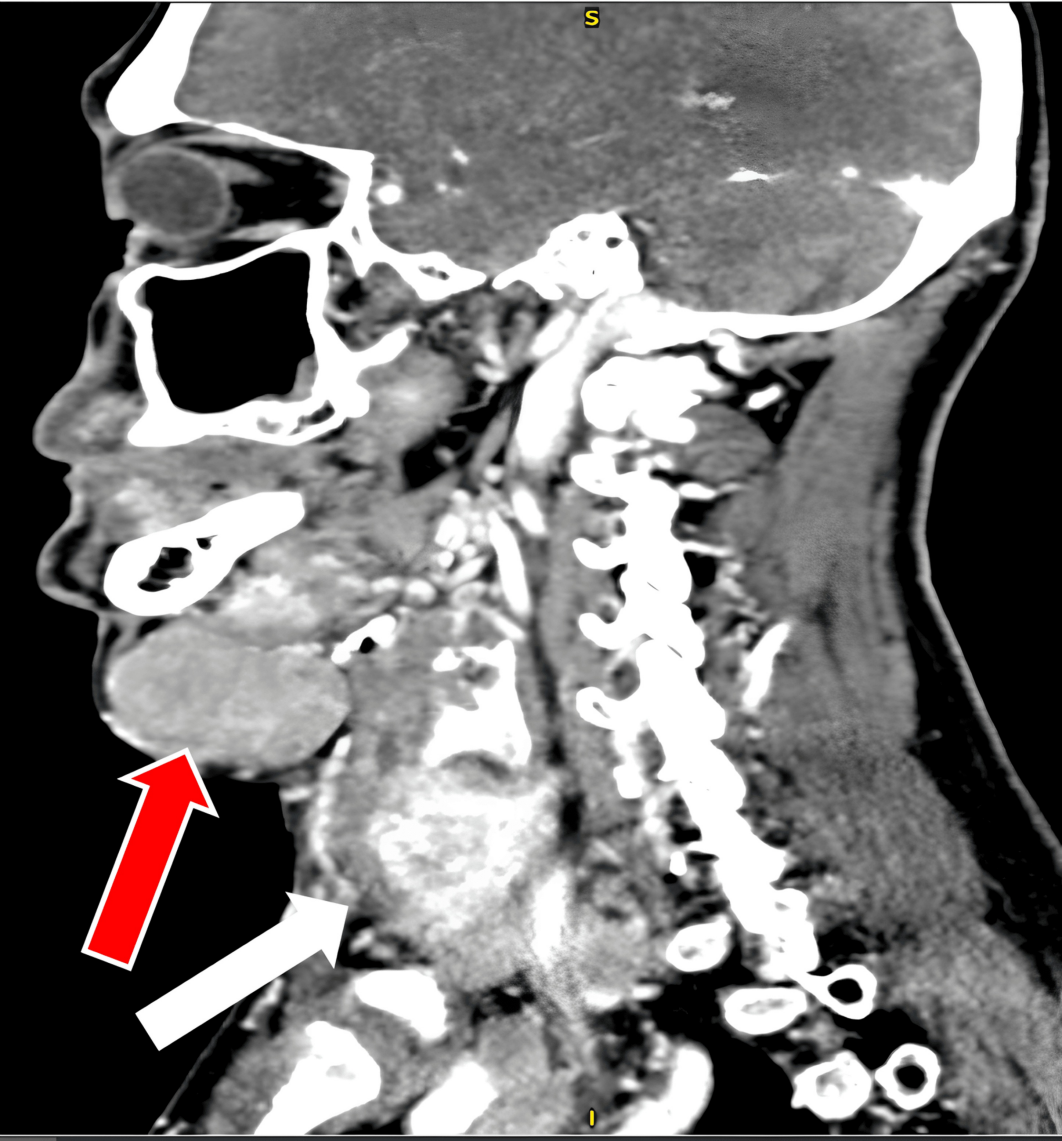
Sagittal view of the computed tomography neck and thorax for this patient. Findings showed bilateral cervical and mediastinal lymphadenopathies with lower cervical and central venous compression. Thyroid gland is heterogeneously enhanced and enlarged, and these lobes are displacing the trachea to the left. Bilateral lung nodules with manubrial lytic lesion are suspicious of metastasis. Red arrow points toward patient's submental cervical lymphadenopathy. White arrow points toward patient's thyroid region.
